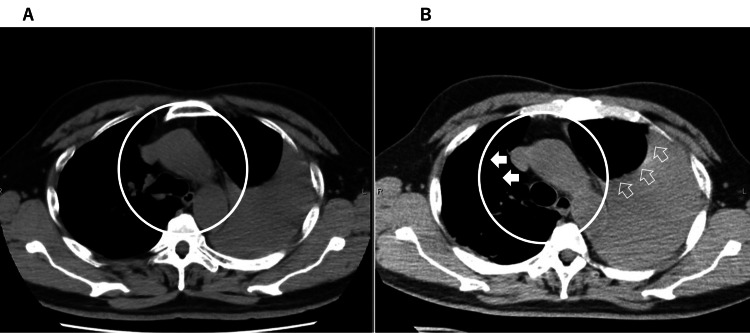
CT scan showing a slightly bigger hemothorax with a mediastinal shift performed at our hospital (two hours after obtaining Figure 2). A: CT scan performed at the referring hospital (taken at almost the same time as Figure 1). B: CT scan performed at our hospital.
